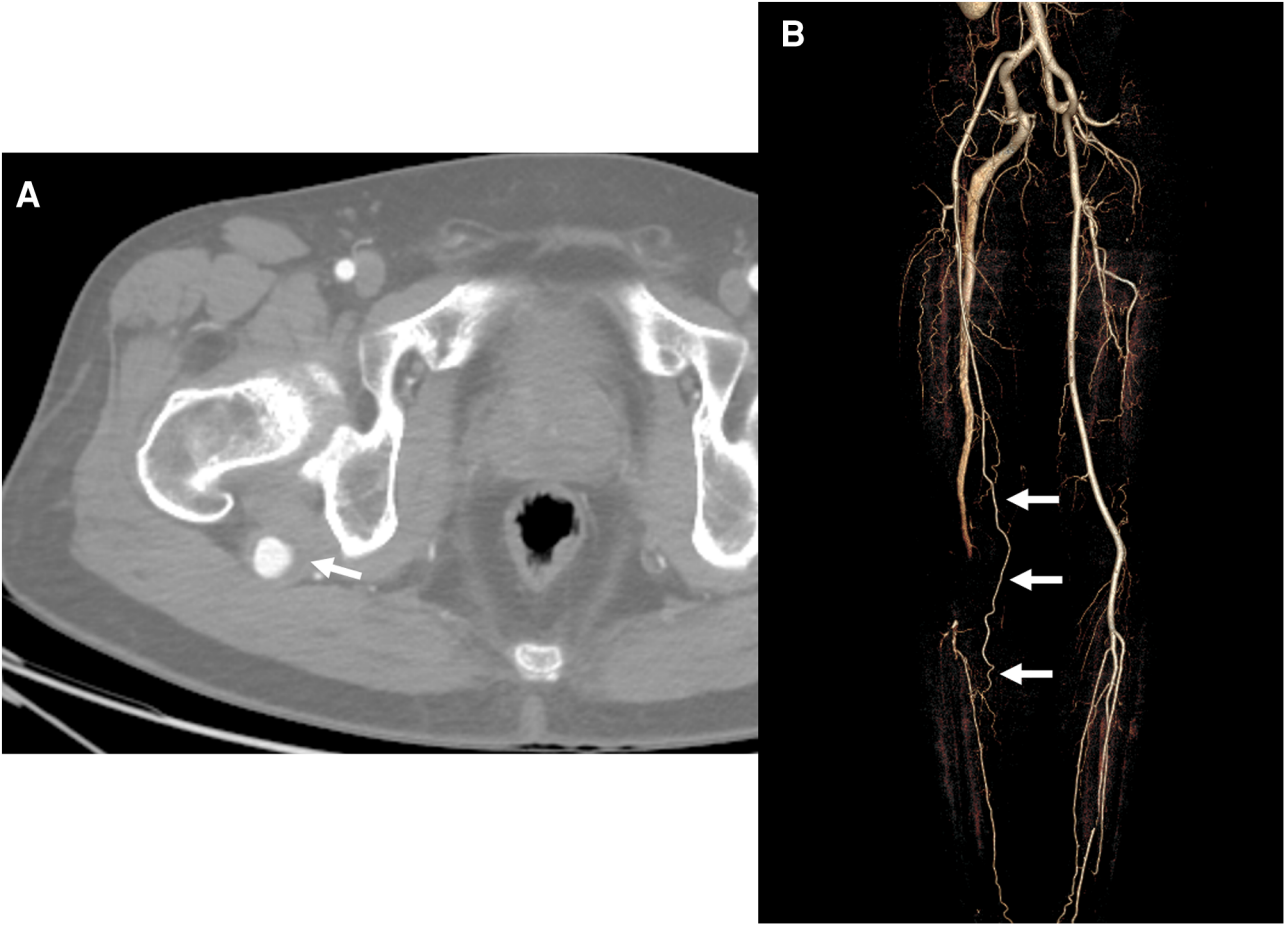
Initial computed tomography angiography (CTA) of the second case. (A) Axial image of the CTA revealed an aneurysm with a diameter of 22 m and a mural thrombus of the persistent sciatic artery (PSA) (*arrow*) at the posterior aspect of the greater trochanter of the femur. (B) Three-dimensional reconstruction image revealed a collateral flow (arrows) to the calf and foot from the incomplete superficial femoral artery and occluded distal popliteal artery connected with the PSA.
